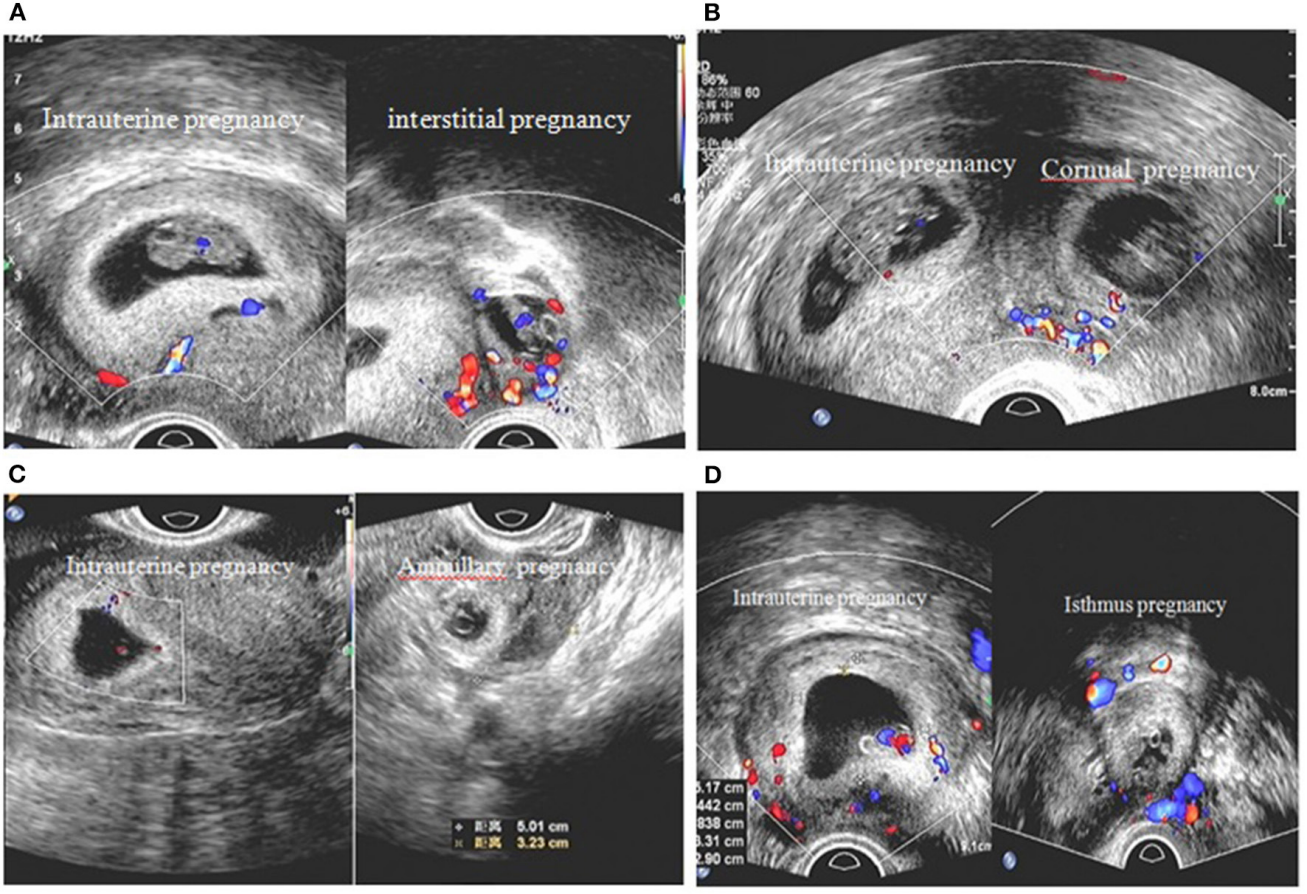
Ultrasound diagnosis of intrauterine pregnancy and location of ectopic pregnancy. Heterotopic pregnancy with different locations: (A) intrauterine pregnancy and left interstitial pregnancy (B) intrauterine pregnancy and right cornual pregnancy (C) intrauterine pregnancy and left fallopian ampullary pregnancy (D) intrauterine pregnancy and left fallopian isthmus pregnancy.
